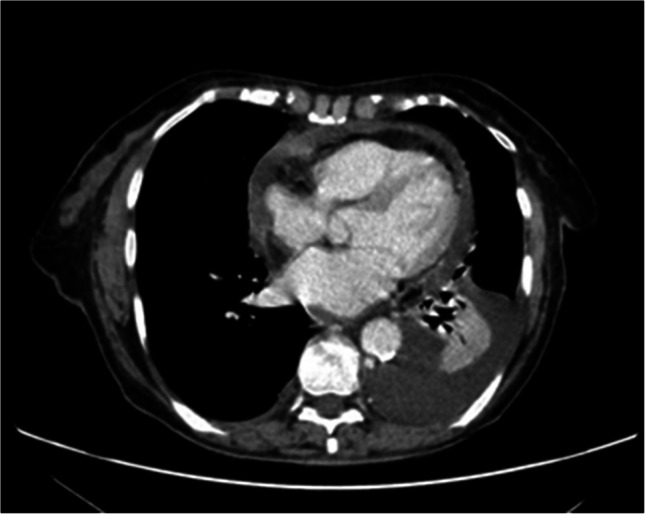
Chest CT showing pleural and pericardial effusion
